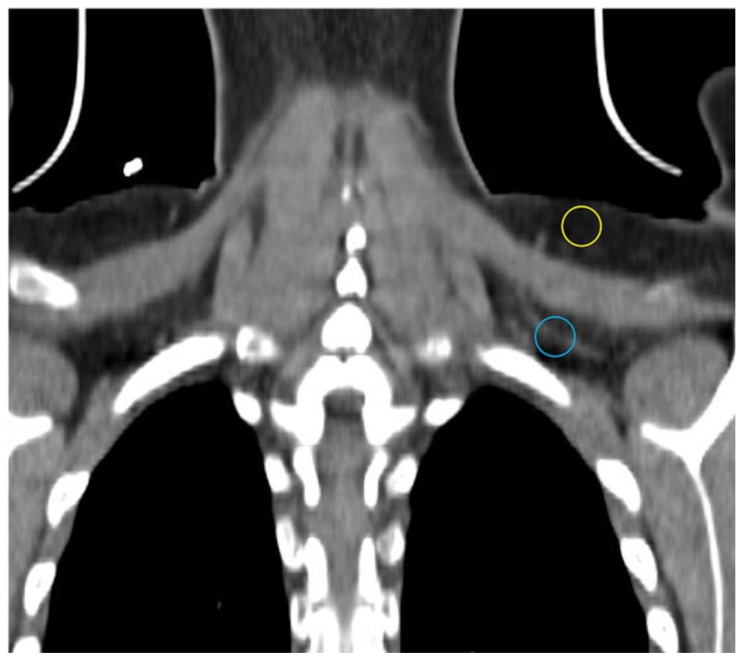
CT showing different density and vascularity of BAT versus WAT. Subcutaneous WAT with a darker appearance on conventional display and fewer vessels (yellow circle) compared with BAT (blue circle).
